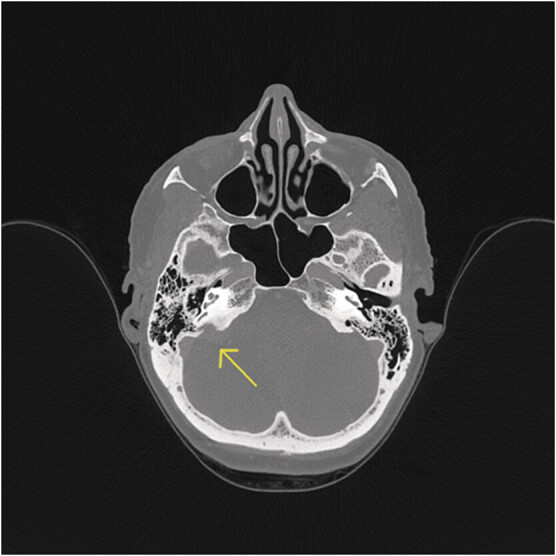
CT scan showing bony changes in the vestibular aqueduct.
